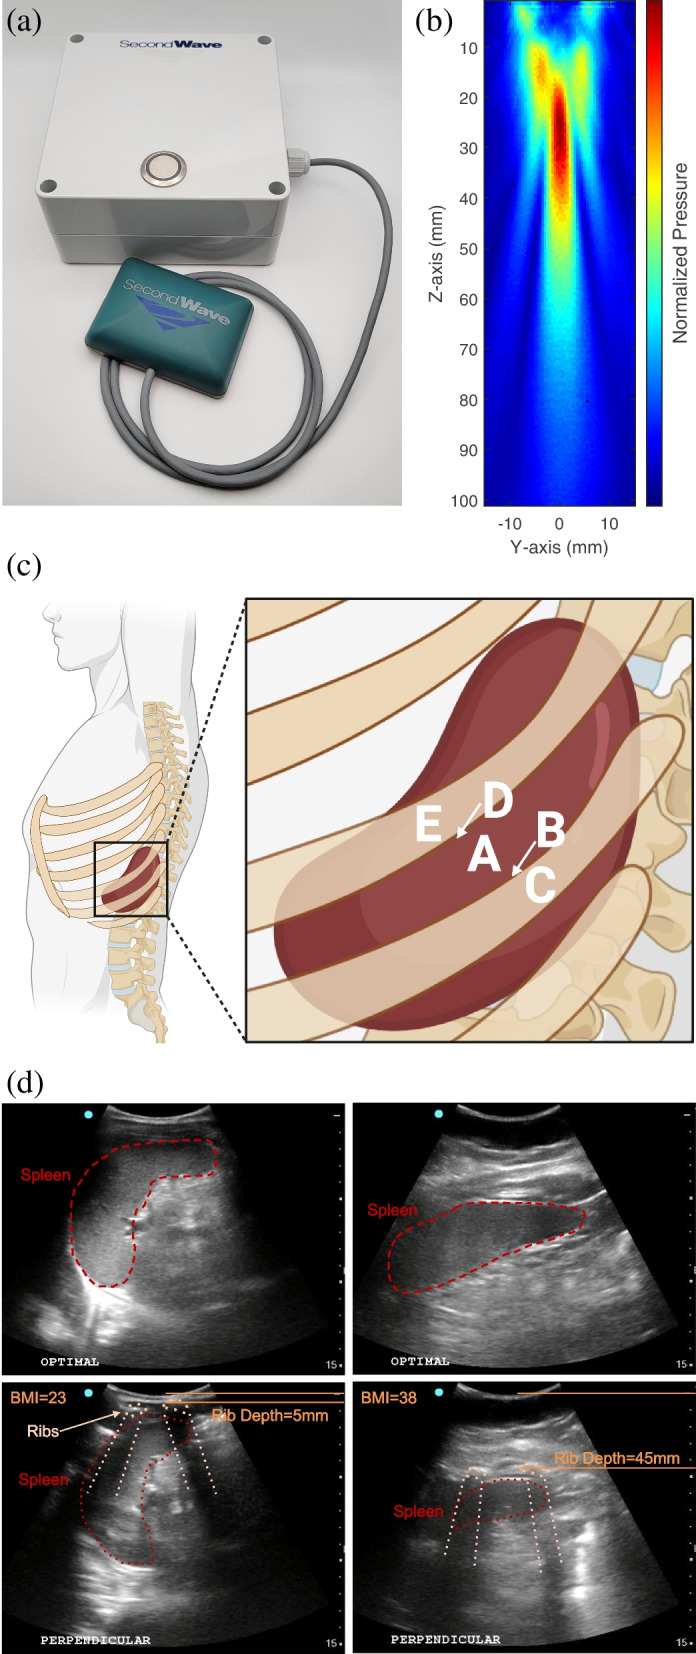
Detection of ribs over the spleen. a Therapeutic ultrasound device from SecondWave Systems. b Pressure profile of ultrasound. c Anatomical locations where rib echo signals were acquired: A, between ribs; B, partially over first rib; C, directly over first rib; D, partially over second rib; E, directly over second rib. Created with BioRender.com. d Ultrasound images collected with Sonosite Edge II: top row, transducer probe parallel to the ribs; bottom row, perpendicular to the ribs such that ribs are visible. Left column of images shows low BMI example (BMI = 23) and right column shows high BMI example (BMI = 38)
